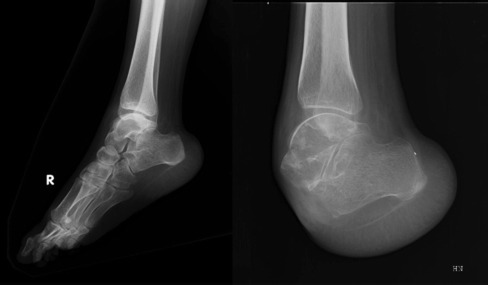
Preoperative and postoperative radiographs of right foot.
